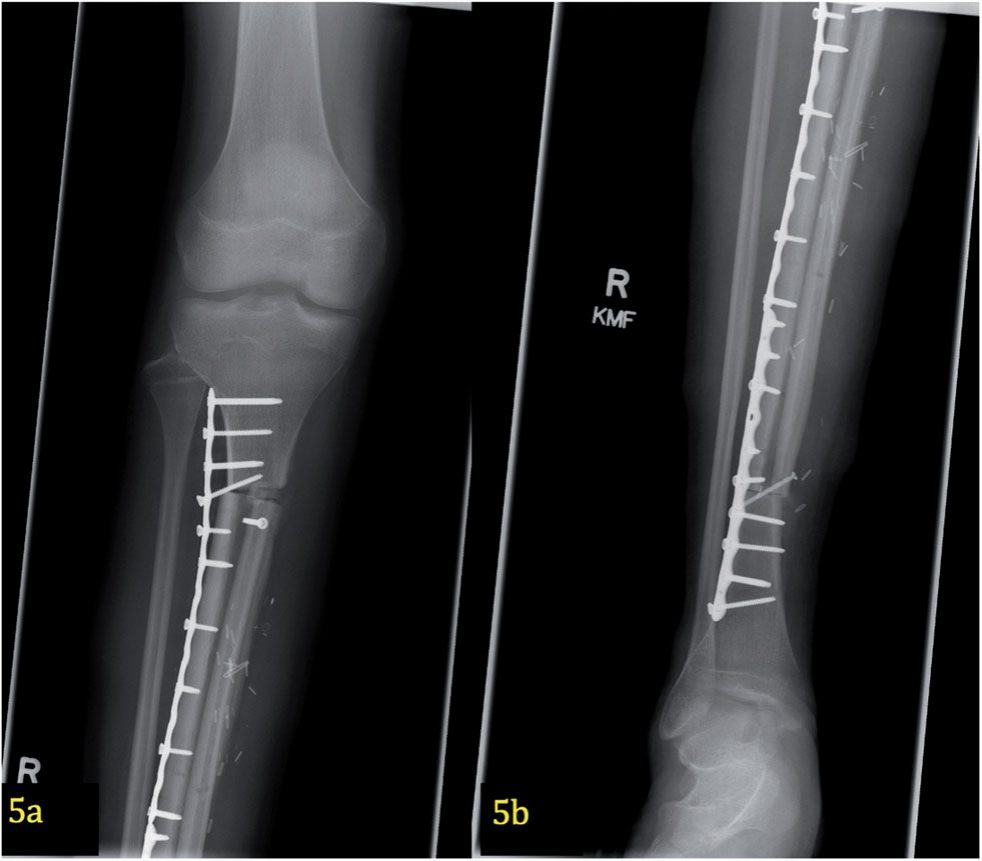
(a,b) Post-operative X-ray of free microvascular fibular graft. At 29 months postoperatively, proper alignment of the hemi-tibia allograft and free microvascular fibular graft can be seen in the proximal (5a) and distal (5b) portions of the tibia. This AP image shows two plates with screws spanning the entire defect.
